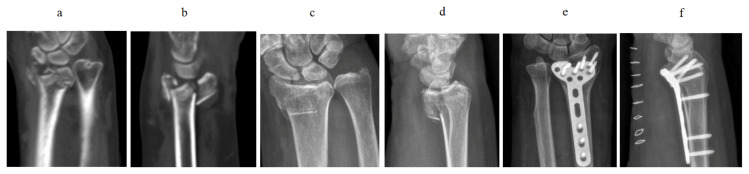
Patients whose pronator quadratus was preserved. a, b) preoperative CT scan showed a distal radius fracture of type C2 before the fixation operation. c, d) Preoperative X-Ray showed a distal radius fracture of type C1. e) One week after operation, the anterior and posterior films were displayed. f) One week after operation, lateral radiography was shown.
